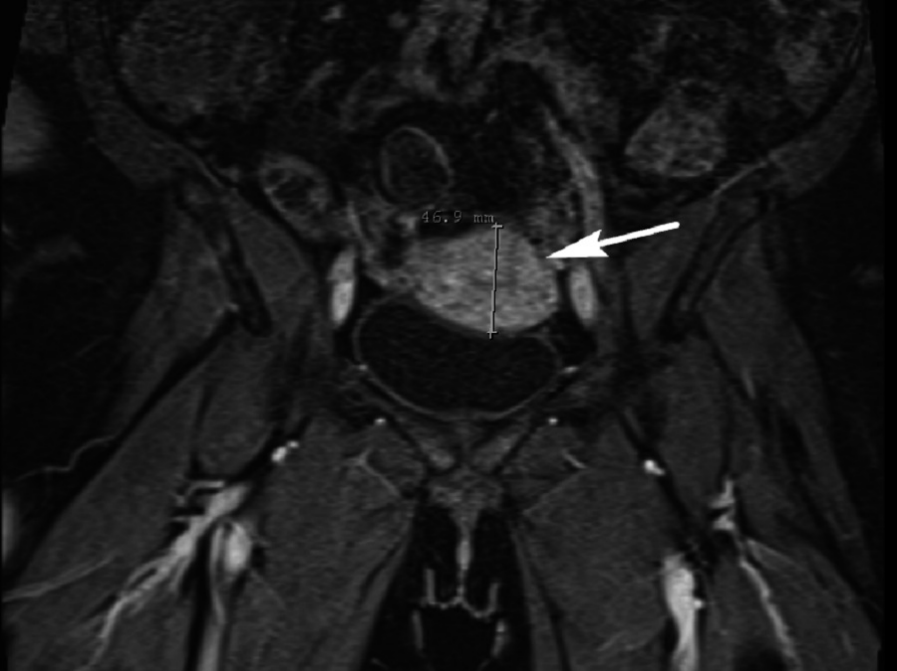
Abdominal magnetic resonance imaging (MRI) Abdominal MRI shows a 4.5 cm concentric apple core tumor (arrow) in the wall of the proximal to mid-rectum region with only a small residual patent lumen present.
